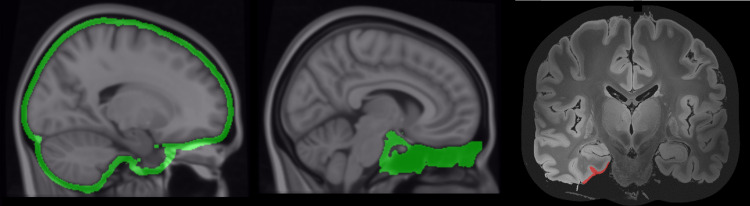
Custom ROI for meningeal (left), and sinus (center) uptake used for our analysis overlaid on the MNI-152 brain template. The ROI for the ERC (right) was segmented on the ultra-high-resolution MRI shown (discussed inSection 2.4).
